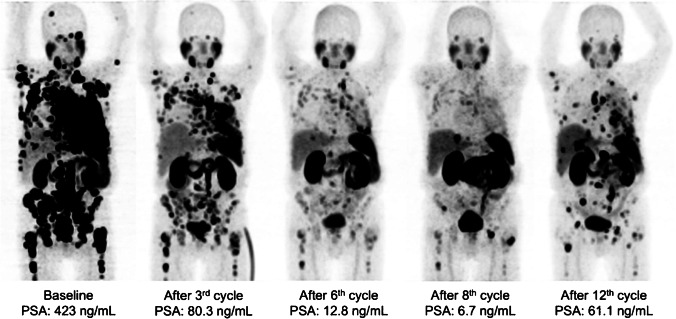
From left to right: MIP [^68^Ga]Ga-PSMA-11 PET images of a patient with disseminated bone and lymph node metastases, pleural carcinosis along with infiltration of the thoracic wall, lymph node metastases, and local disease responding to the initial cycles of extended treatment. [^177^Lu]Lu-PSMA RLT was discontinued upon progression after 12 cycles (PFS: 21 months)
